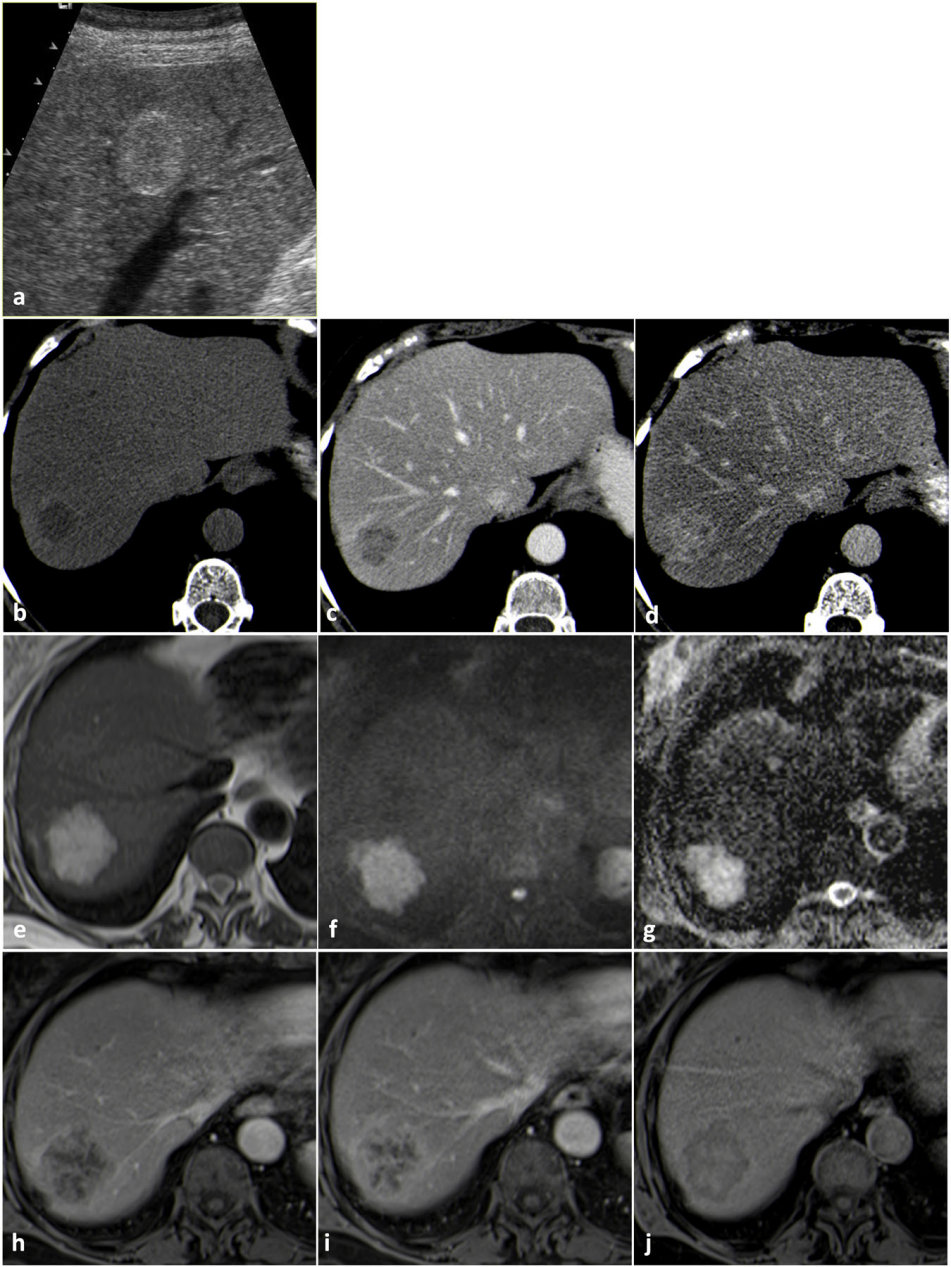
Mucinous colorectal cancer metastasis (biopsy-proven and resected). The lesion appears heterogeneously hyperechoic on B-mode US (a). On CT the lesion appears markedly hypodense in the native phase (b) and shows progressive enhancement in the portal venous (c) and equilibrium (d) phase. On MRI the lesion appears markedly hyperintense on T2-weighted images (e), determining shine through artifact on high b-value DWI (f) without true diffusion coefficient restriction on ADC map (g). The lesion shows peripheral enhancement in late arterial phase (h) with progressive centripetal filling in portal venous phase (i). In hepatobiliary phase (j) the central component shows contrast material retention
